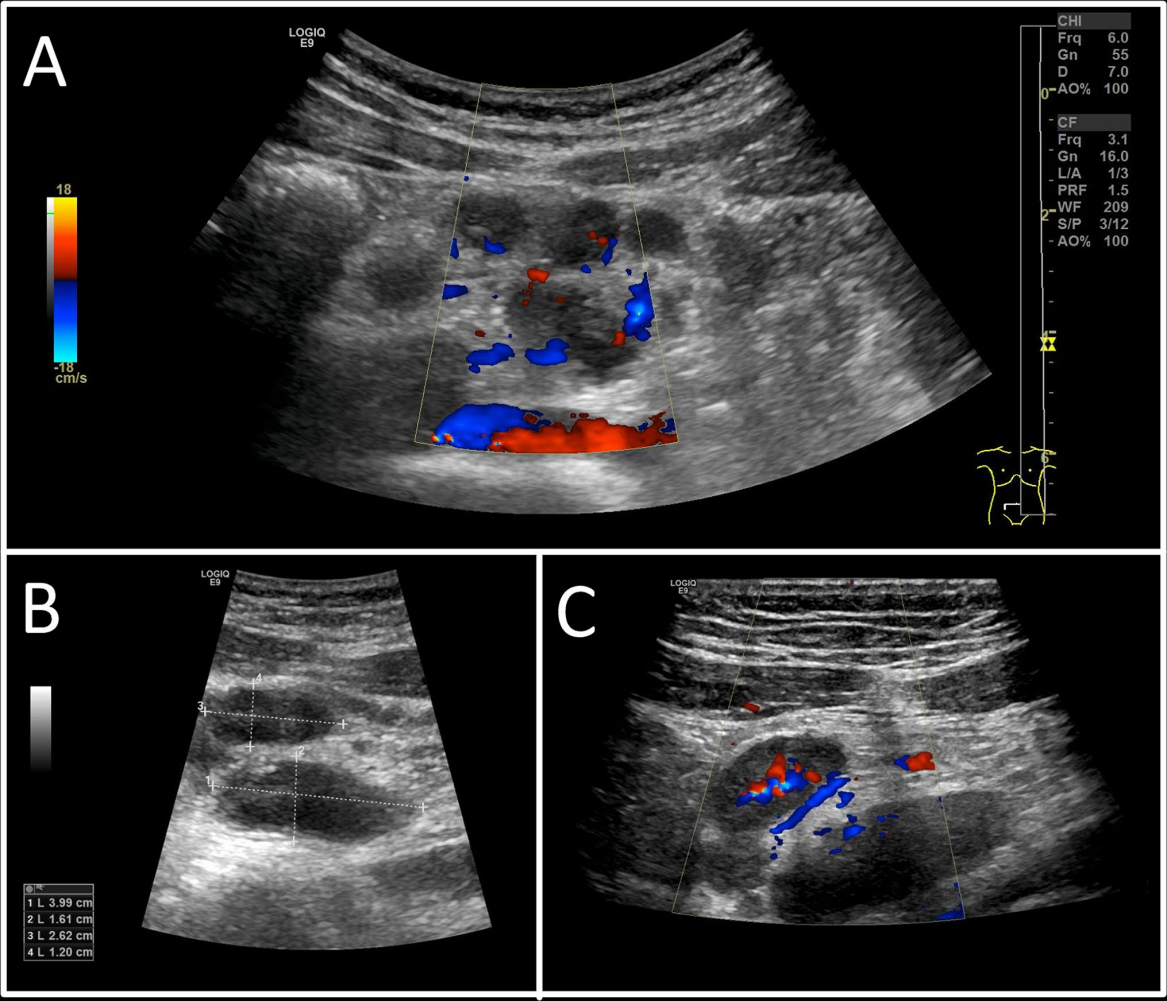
Abdominal ultrasound examination reveals multiple enlarged mesenteric lymph nodes in the right lower quadrant. a Hypoechoic lymph nodes surrounded by hyperechoic mesenteric tissue. b Diameters of the two largest oval shaped lymph nodes (max. diameter 4 cm). c Hilus perfusion of the lymph nodes depicted by duplex ultrasound
